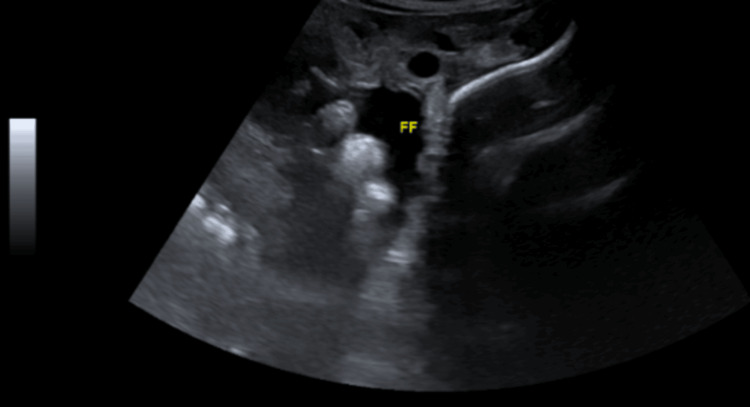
Abdominal ultrasound showing free turbid peritoneal fluid
